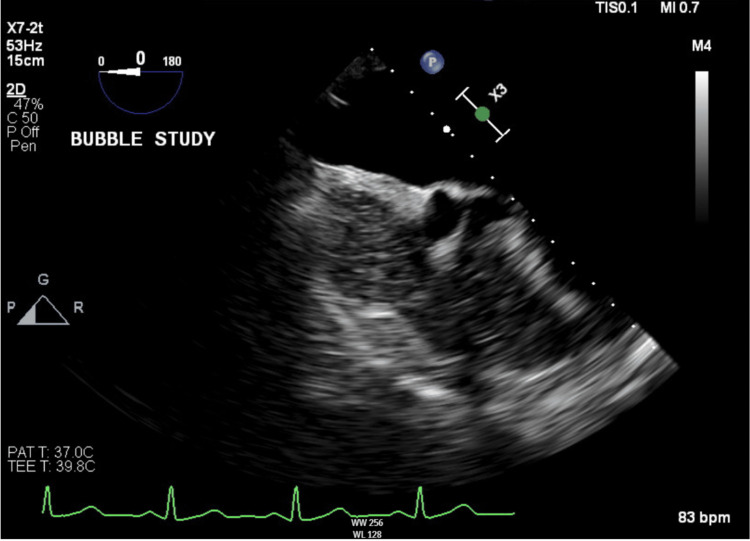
Normal transesophageal echocardiography with bubble study.
